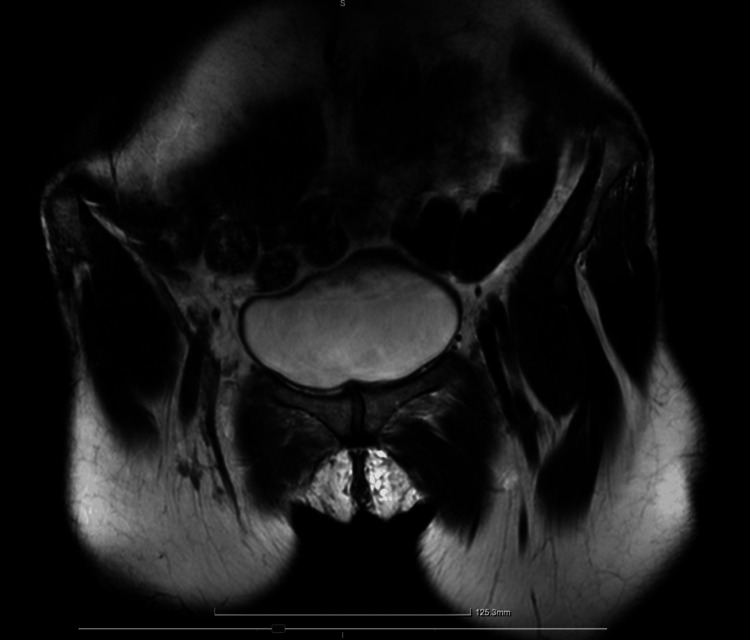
Magnetic resonance imaging (MRI) on postoperative day 4 demonstrating no evidence of periurethral injury or hematoma T2 sequence MRI without contrast enhancement
